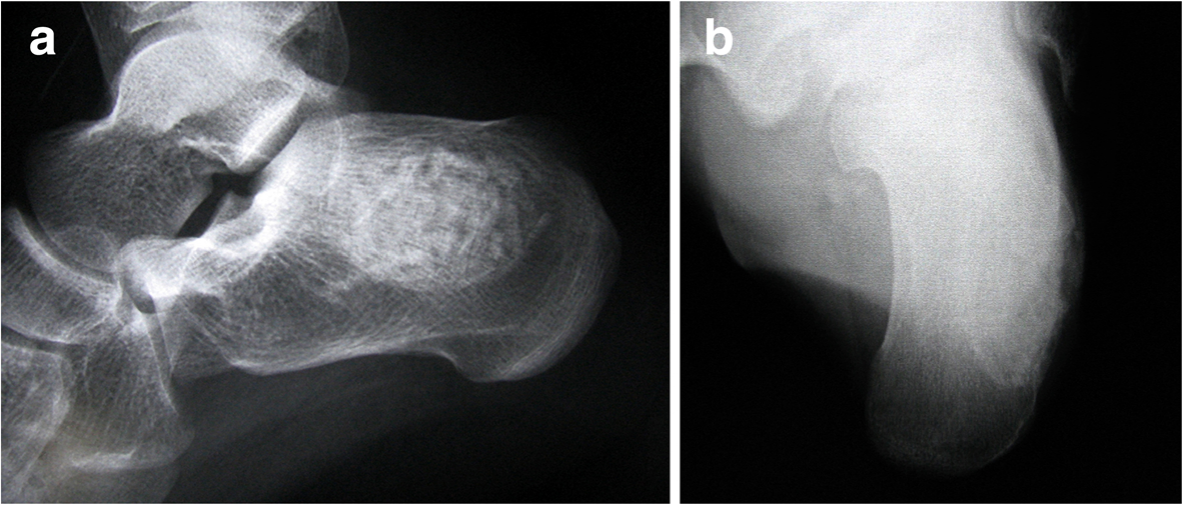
Post-operation radiograph of the calcaneum. a Lateral image and (b) axial image show the surgical cavity is filled with bone grafts
